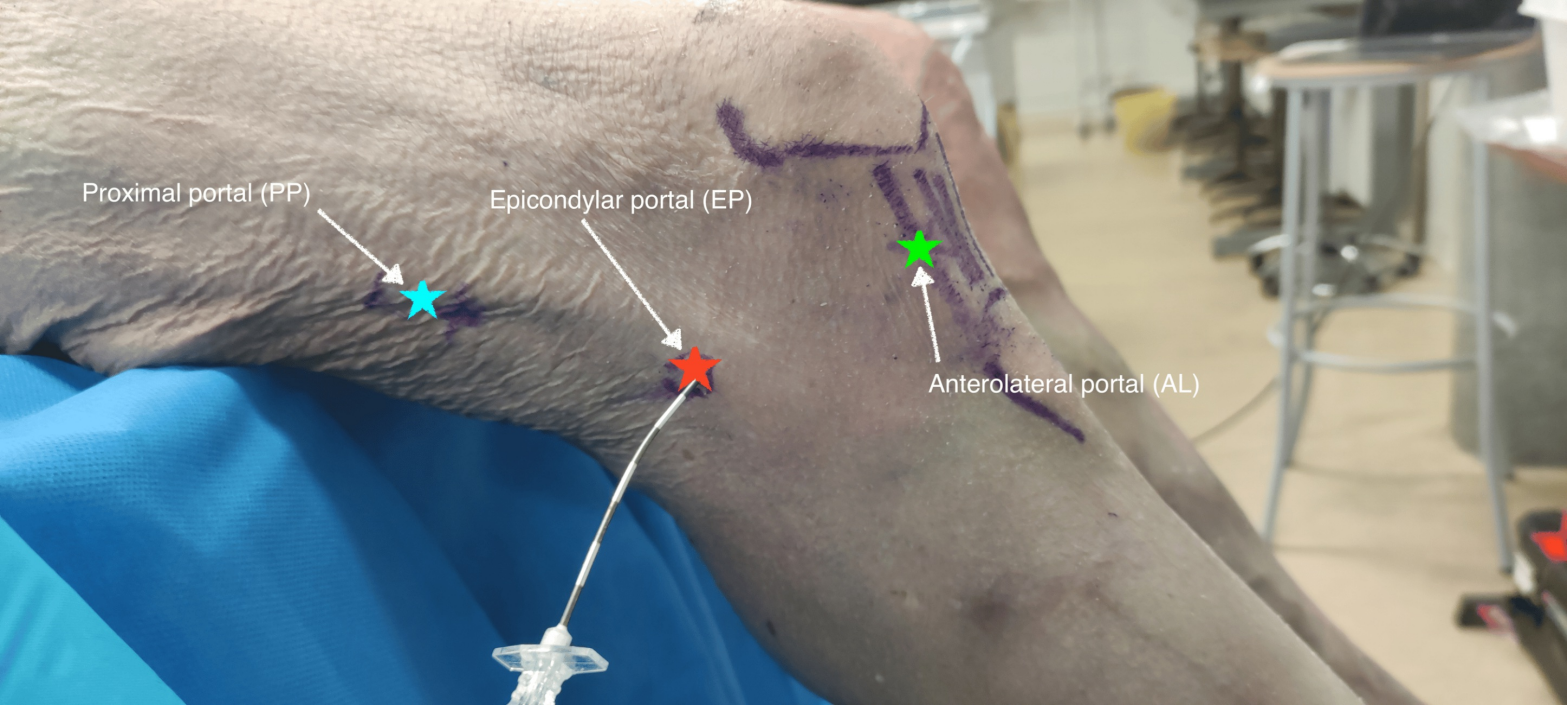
Marked endoscopic portals Three endoscopic portals were established: one at the level of the LE (EP), one located 5 cm proximally along the thigh axis (PP), and a standard AL. These portals were drawn and aligned on the skin. The EP was identified using ultrasound guidance. Knee flexion could be adjusted intraoperatively to optimize alignment of the three portals. AL, anterolateral portal; EP, epicondylar portal; LE, lateral epicondyle; PP, proximal portal
